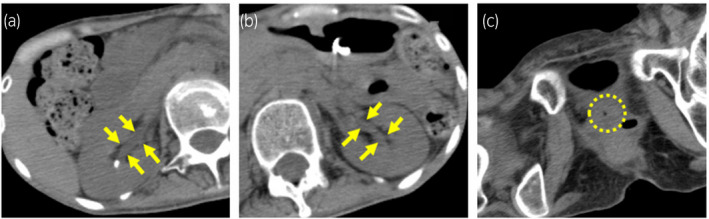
CT performed 6 months after the operation. (a,b) The arrows revealed that bilateral hydronephrosis disappeared. (c) The circle revealed that calculus was not detected at the same slice as Figure 1a.
